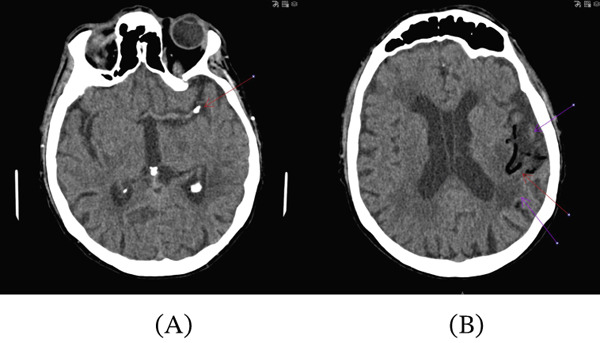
Computed tomography (CT) of the brain in soft tissue window (A and B), performed within 1 h after the event, showing the dense artery sign (red arrow in A) corresponding to the earlier known occluded segment of the middle cerebral artery with surrounding hypodensities (purple arrows in B) representing demarcation of ischemic changes. It also reveals the new finding of arterial air embolism along M2 and M3 branches (red arrow in B) of the left middle cerebral artery.
